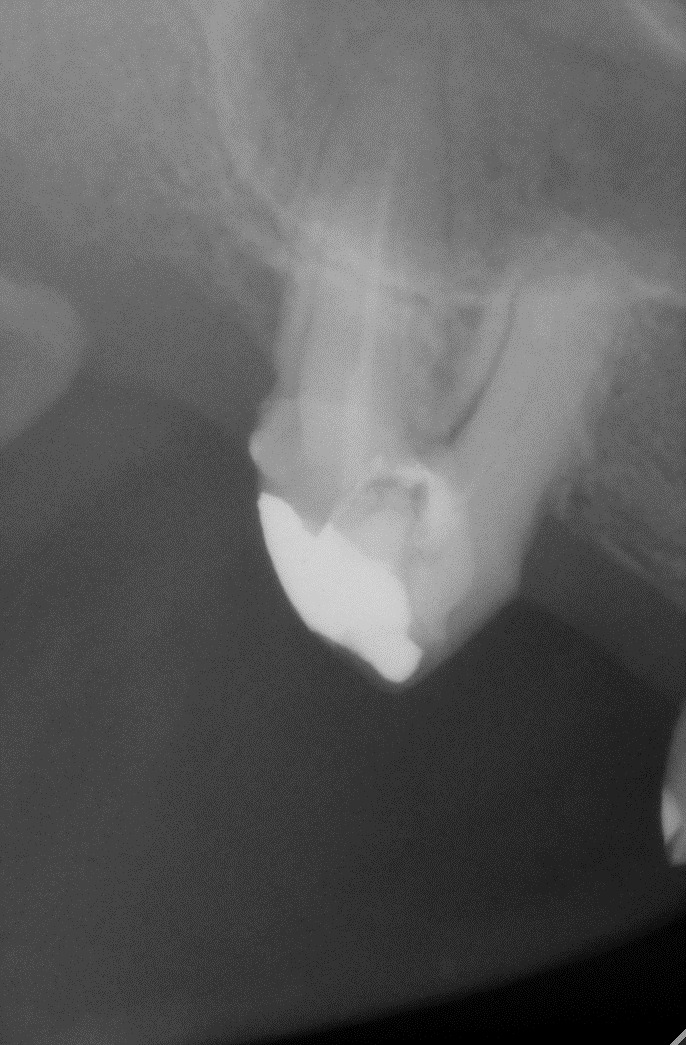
1.6 radiography realized before the treatment; incomplete root canal filling; via falsa in the floor of the pulp chamber incorrect filled; inflammation still present
